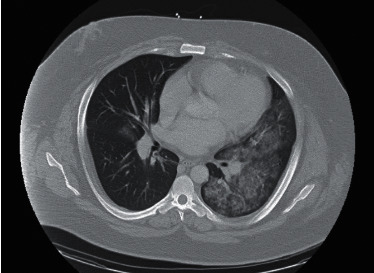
Computed tomographic angiogram of the chest showing bilateral infiltrates more extensive on the left.
